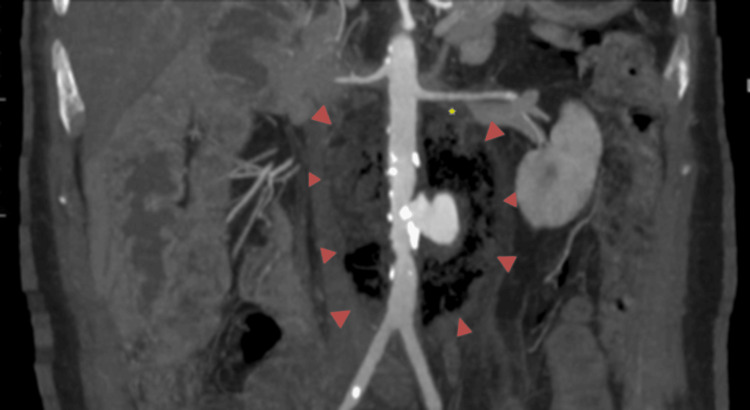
A maximum intensity projection image in the coronal view of the CTA aorta showing the infrarenal periaortic collection (red arrowheads) with mycotic aneurysm, in relation to the renal vessels and aortic bifurcation. No clear plane between this collection with the left renal vein. Thrombus seen within this vessel (yellow asterisk). CTA: Computed tomography angiography
